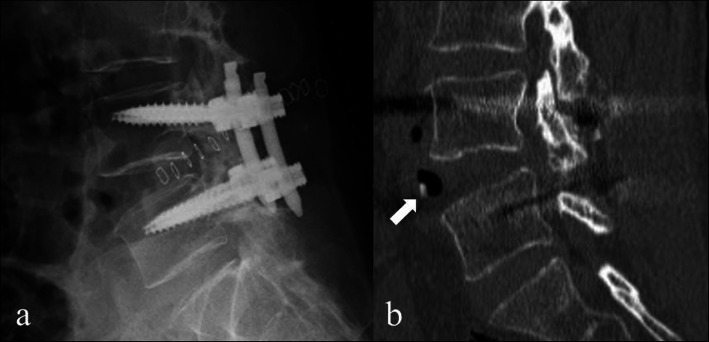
Failure of extreme lateral interbody fusion (XLIF) for a patient with L4 spondylolisthesis. L4–5 fusion surgery, including XLIF, is planned for a 58-year-old woman with L4 spondylolisthesis. However, the anterior longitudinal ligament (ALL) is injured during the XLIF procedure; hence, XLIF is abandoned because it is impossible to fix a cage. Postoperative radiography reveals that the interbody space at the L4/5 level expanded abnormally a. After surgery, a bony endplate fracture (white arrow) associated with the ALL tear is detected on computed tomography b
